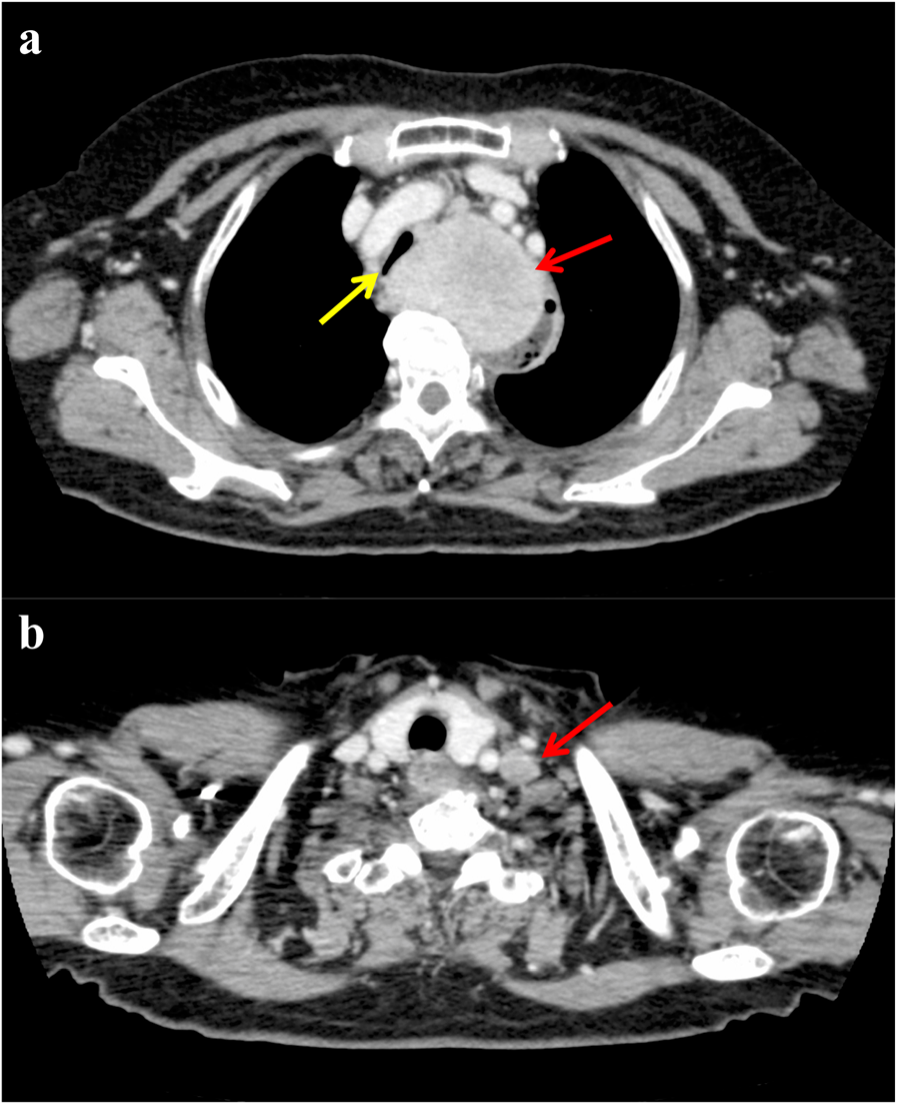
Computed tomography imaging scan. a Red arrow indicates the mediastinal tumor and yellow arrow indicates tracheal stenosis. b Red arrow indicates supraclavicular lymph node enlargement
